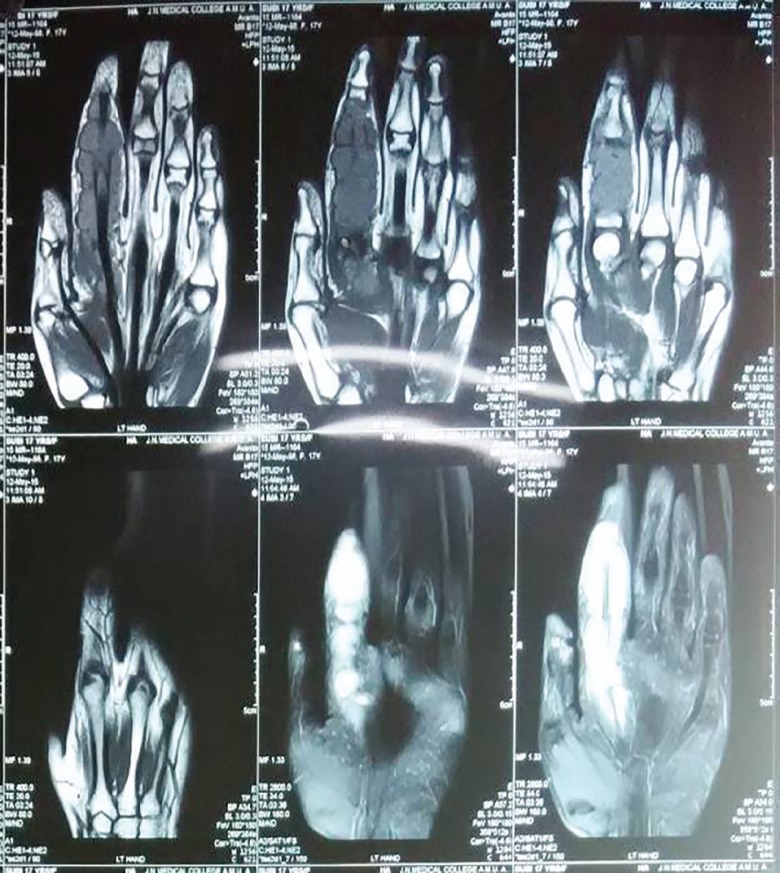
Magnetic resonance image
